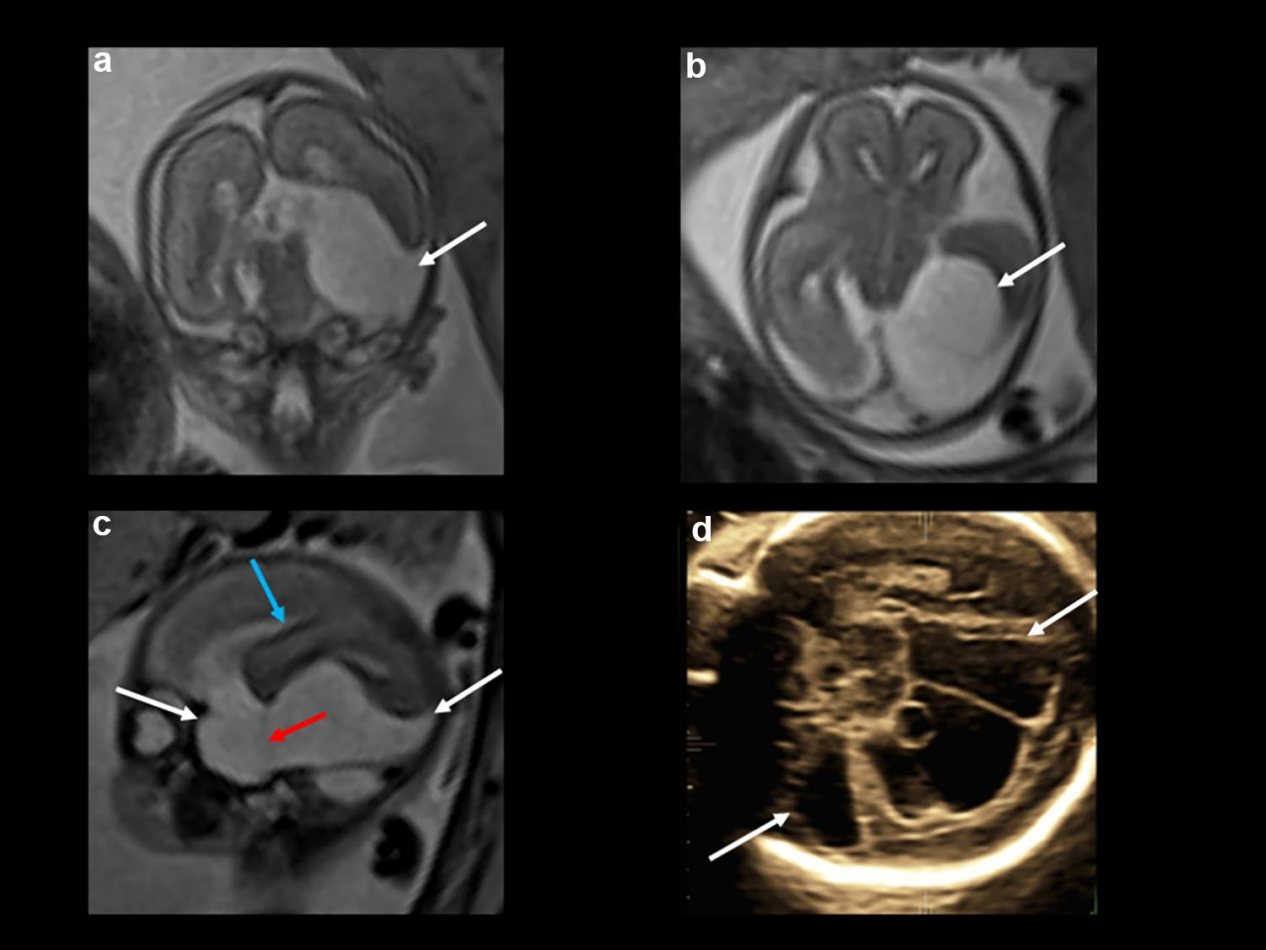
a, b, c Prenatal MRI at 22 weeks of gestational age (T2-weighted) in coronal (a), axial (b), and sagittal (c) orientation (courtesy of Prof. Dr. S. Stöcklein, Ludwig-Maximilians-University, Munich): left-sided supratentorial cystic formation (white arrows) with recognizable septations (c, red arrow) and moderate displacement of the surrounding structures, especially the left temporal lobe (c, blue arrow). d Prenatal ultrasound at 25 + 6 weeks of gestational age: large 4-chambered arachnoid cyst (white arrows) of 7 cm in diameter without any signs of hydrocephalus or brainstem compression (approval of Dr. K. Lato, University Hospital Ulm)
